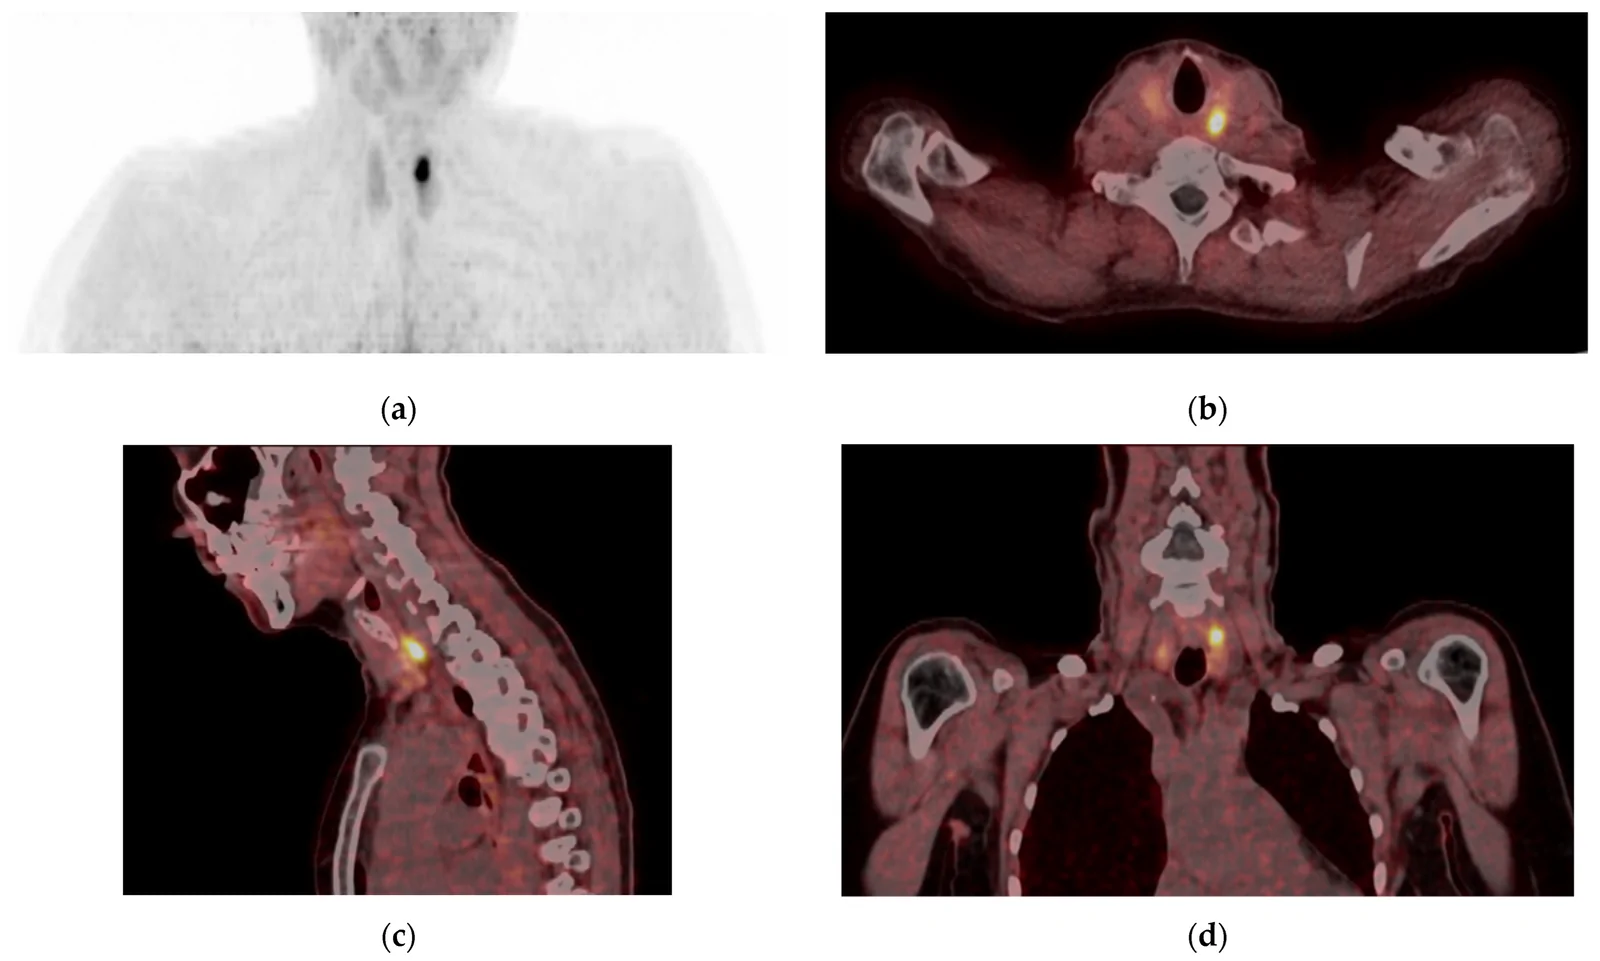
(a) Maximum-intensity-projection (MIP) image of ^68^Ga-Trivehexin PET acquired 30 min post-injection in a 65-year-old woman with hypercalcemia and hyperparathyroidism. Focal intense tracer uptake is seen in the upper-left parathyroid region, corresponding to the suspected parathyroid adenoma; (b) transverse fused ^68^Ga-Trivehexin PET/CT image acquired 30 min post-injection showing a focal area of intense tracer uptake is identified in the upper-left parathyroid region, corresponding to the suspected parathyroid adenoma; (c) sagittal fused ^68^Ga-Trivehexin PET/CT image acquired 60 min post-injection showing persistent focal intense tracer uptake is seen in the upper-left parathyroid region, corresponding to the suspected parathyroid adenoma; (d) coronal fused ^68^Ga-Trivehexin PET/CT image acquired 60 min post-injection showing persistent focal intense tracer uptake is demonstrated in the upper-left parathyroid region, corresponding to the suspected parathyroid adenoma.
